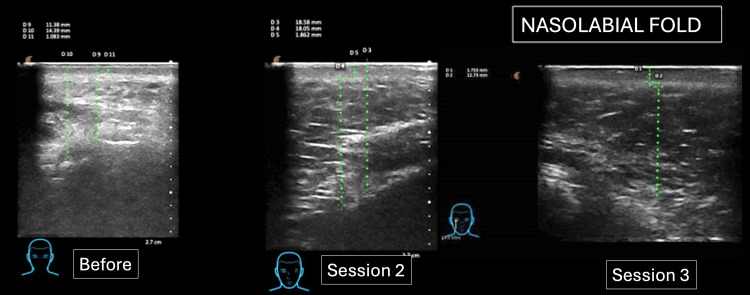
Ultrasound evaluation of the nasolabial fold of a 42-year-old patient, showing the SLEB in the upper area along the dotted green line and its progression over the three sessions. SLEB: subepidermal low-echogenic band.
